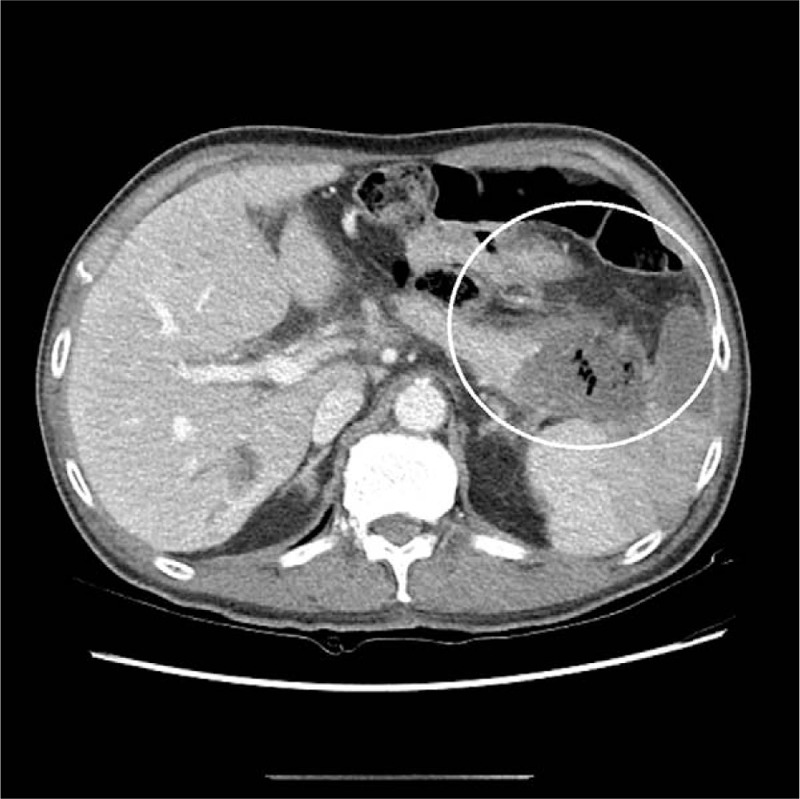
Abdominal CT. A marked fluid collection between pancreatic tail and stomach (white circle). CT = computed tomography.
